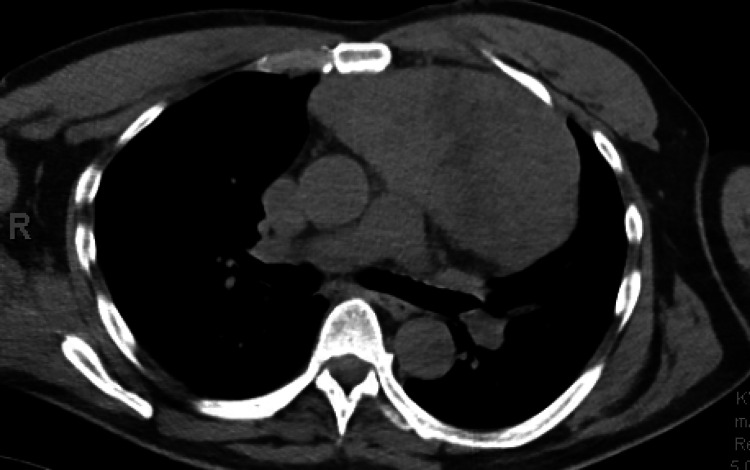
Chest CT showing an anterior mediastinal mass prior to thymectomy
